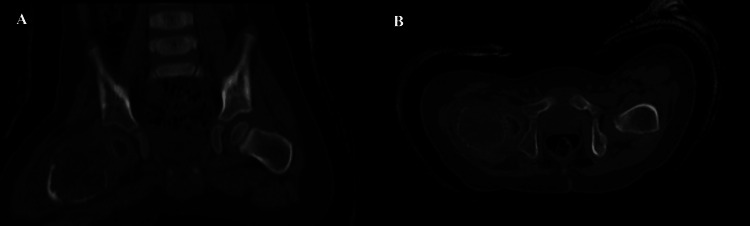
CT coronal (A) and axial (B) sections show a 49x36 mm hypodense cystic expansile lesion with a sclerotic margin in the proximal metaphysis of the right femur
